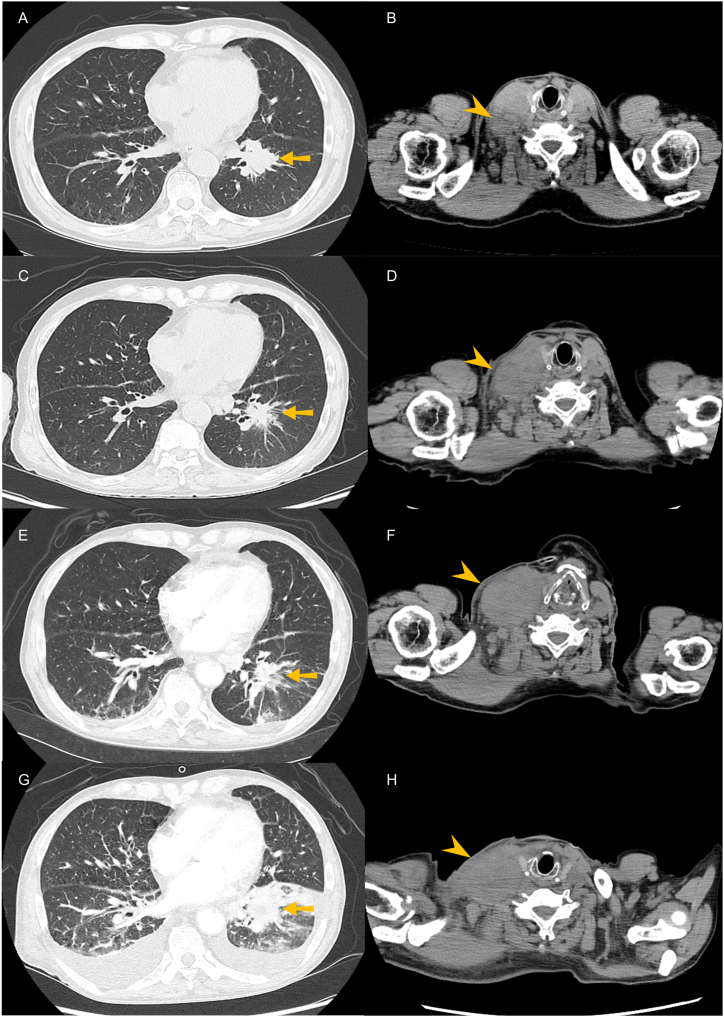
Computed tomography images of lung cancer. Computed tomography revealed the primary tumor in the left lower lobe of the lungs (arrows) and right cervical lymph node metastasis (arrowheads) at diagnosis (A, B). Following treatment with dabrafenib and trametinib, the primary tumor shrank, whereas the cervical lymph node metastasis was enlarged (C, D). At admission because of multiple organ infarction, the primary tumor remained controlled, but the cervical lymph node metastasis had progressed (E, F). Despite second-line treatment with pembrolizumab, the disease further progressed (G, H).
